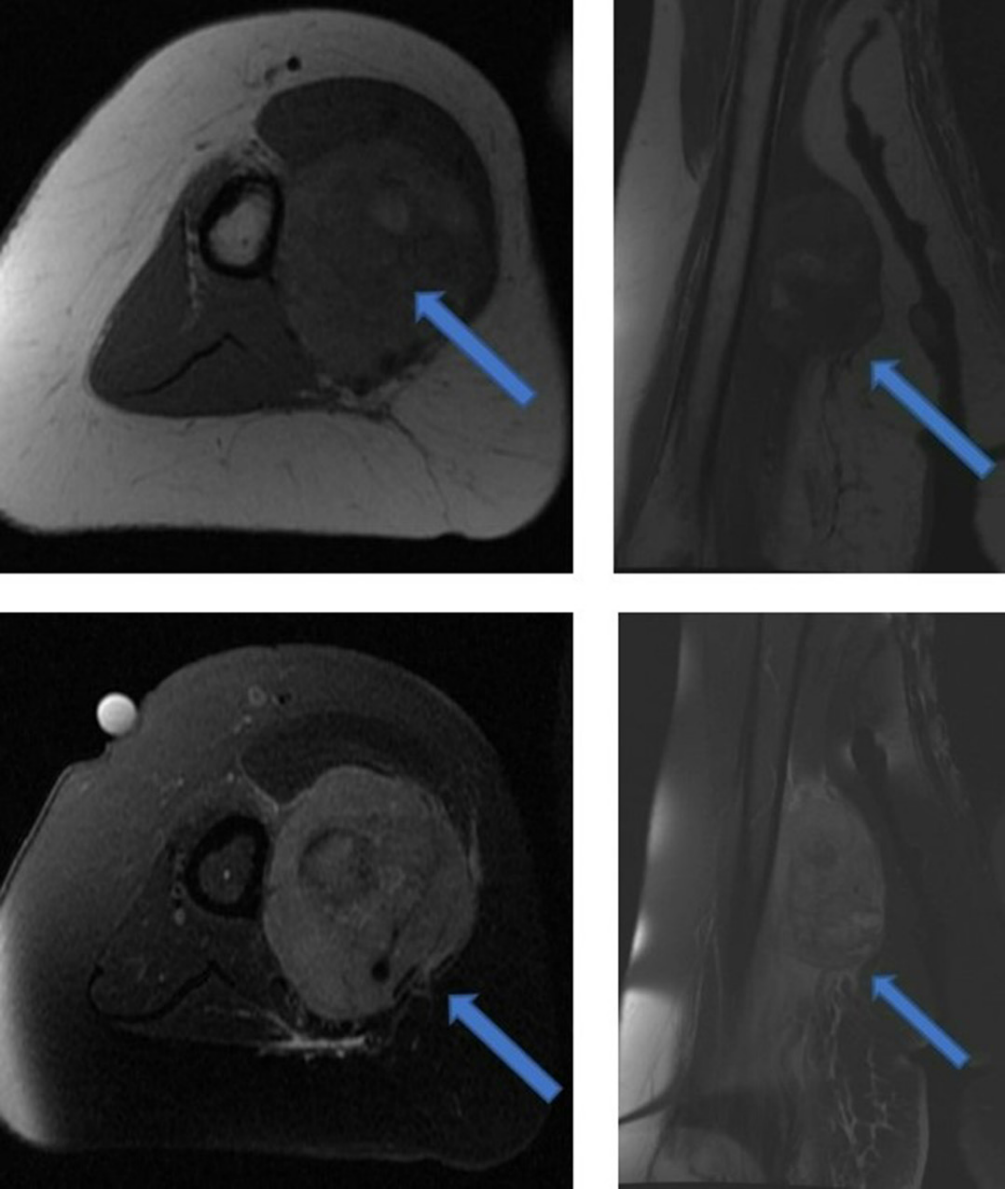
MRI of right upper arm lesion (arrows). Figure 2a and b are *T_1_
